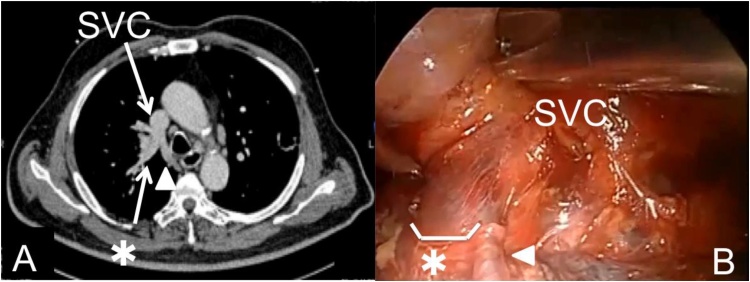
(A) Thoracic computed tomography image showing the right upper pulmonary vein draining into the SVC. (B) Intraoperative photograph showing the PAPVC. Asterisk (*): PAPVC draining into SVC, arrowhead: azygos vein. PAPVC: partial anomalous pulmonary venous connection, SVC: superior vena cava.
